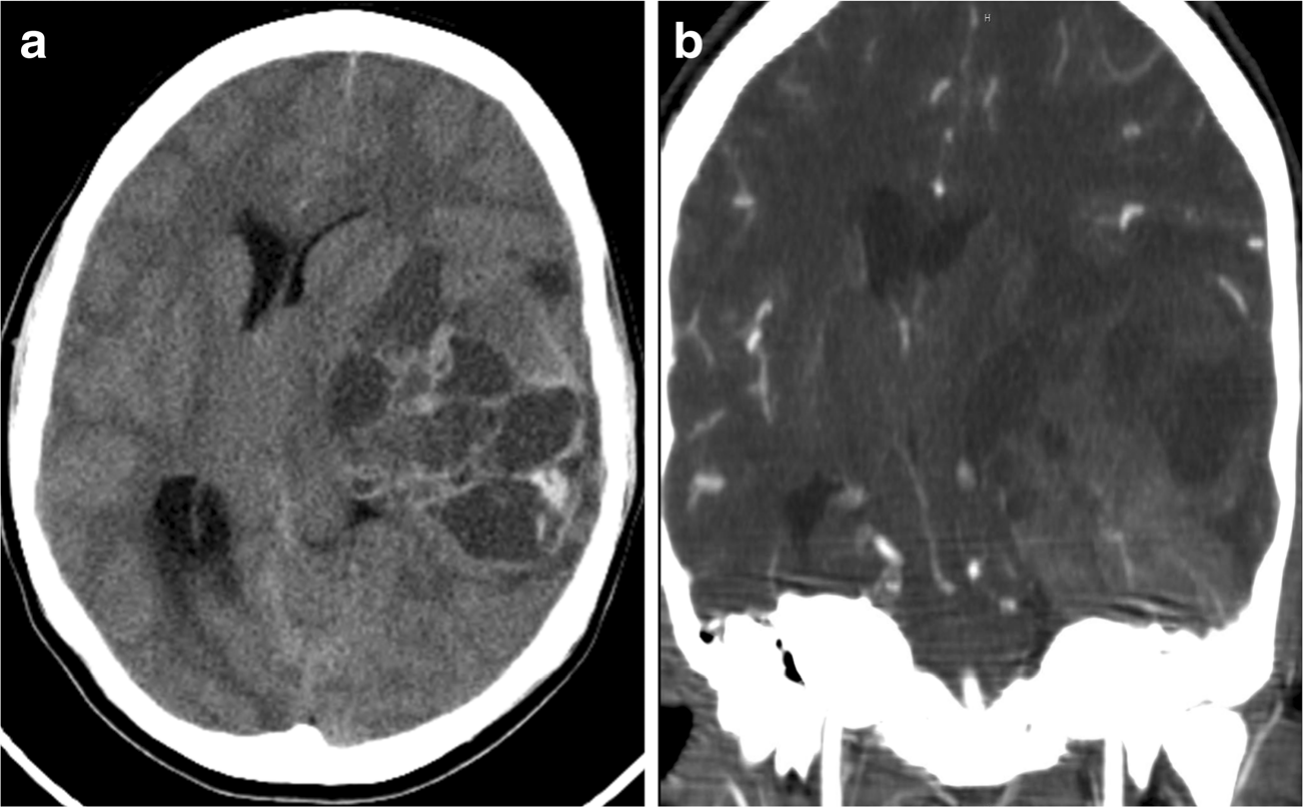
a Images from Case 1 of a 16-year-old female presenting with a left temporal lobe tumor. Non-contrast axial CT. b Post-contrast coronal CT demonstrating bony involvement along the intracranial surface of the inferior left temporal bone
